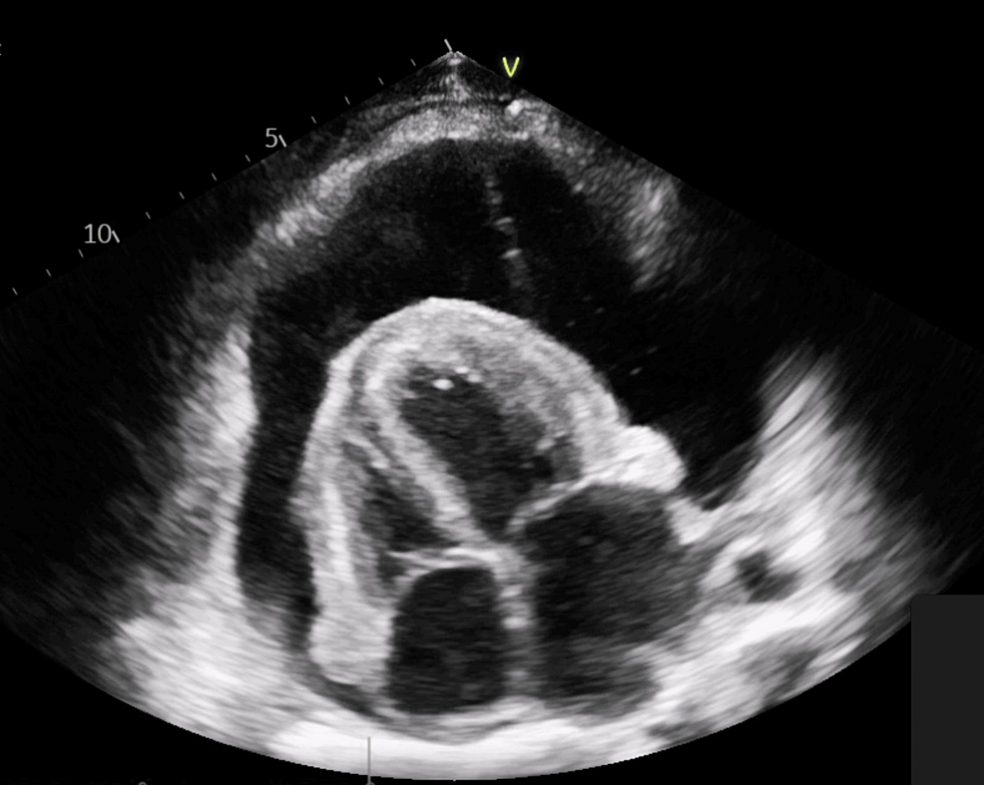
Standard echocardiographic views are used to assess a pericardial effusion. As seen in the figure above, the pericardial effusion is impeding normal myocardial contractility. The emergent transthoracic echocardiography (TTE) demonstrated a large pericardial effusion measuring 5.4 cm posterior to the left ventricle.
